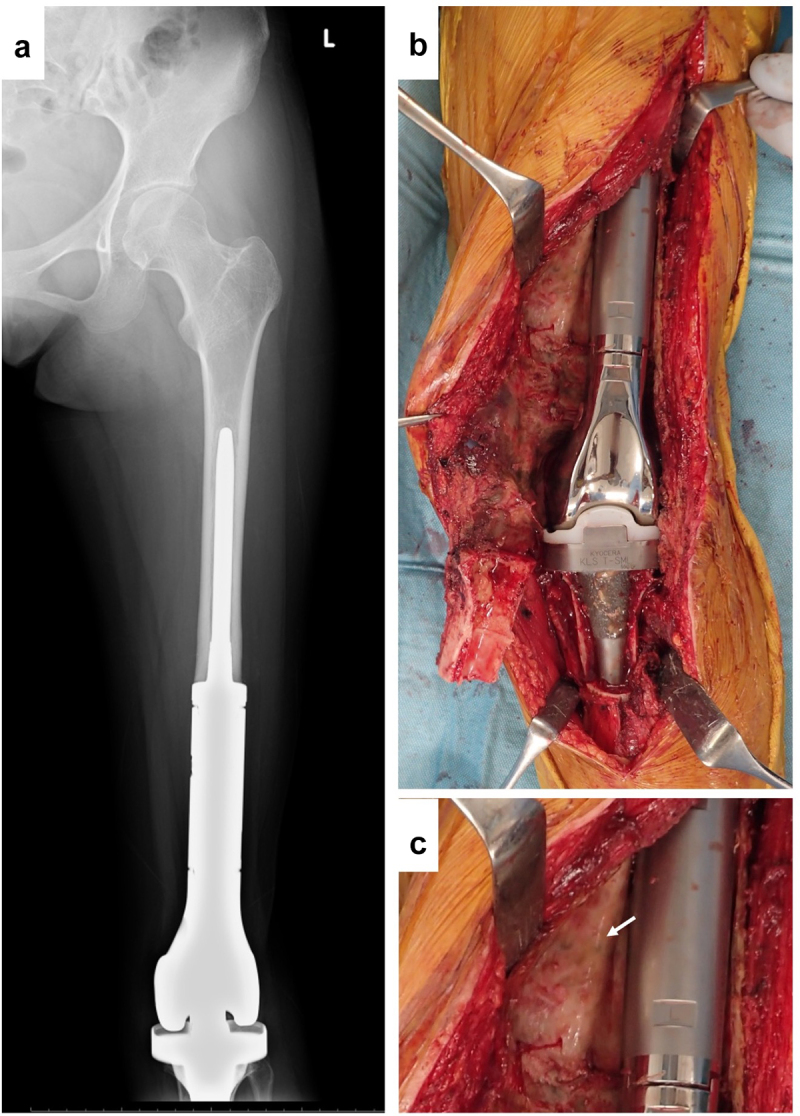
Revision by KMLS (Kyocera Modular Limb Salvage System). (a) X-ray. (b) Intraoperative picture. (c) Soft tissue adjacent to metal surface.
